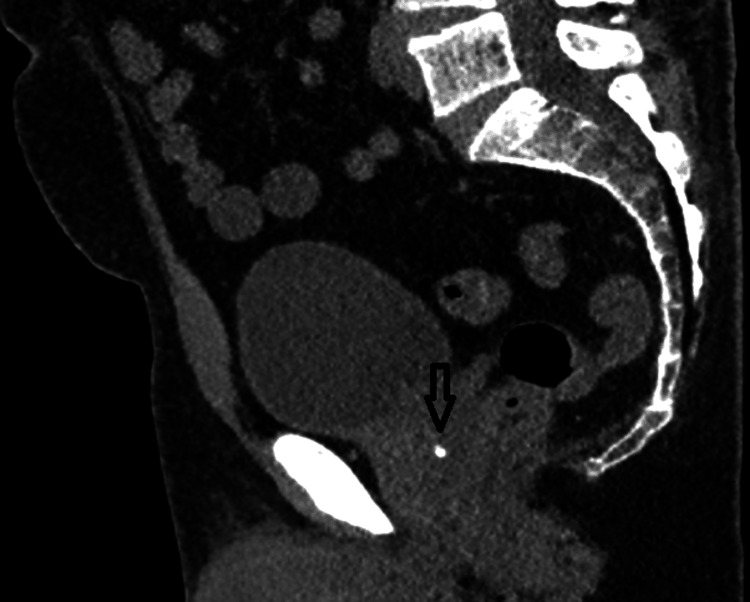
Pre-procedure low-dose CT (sagittal) demonstrating calcification (arrow) within the planned beam path (original image).
